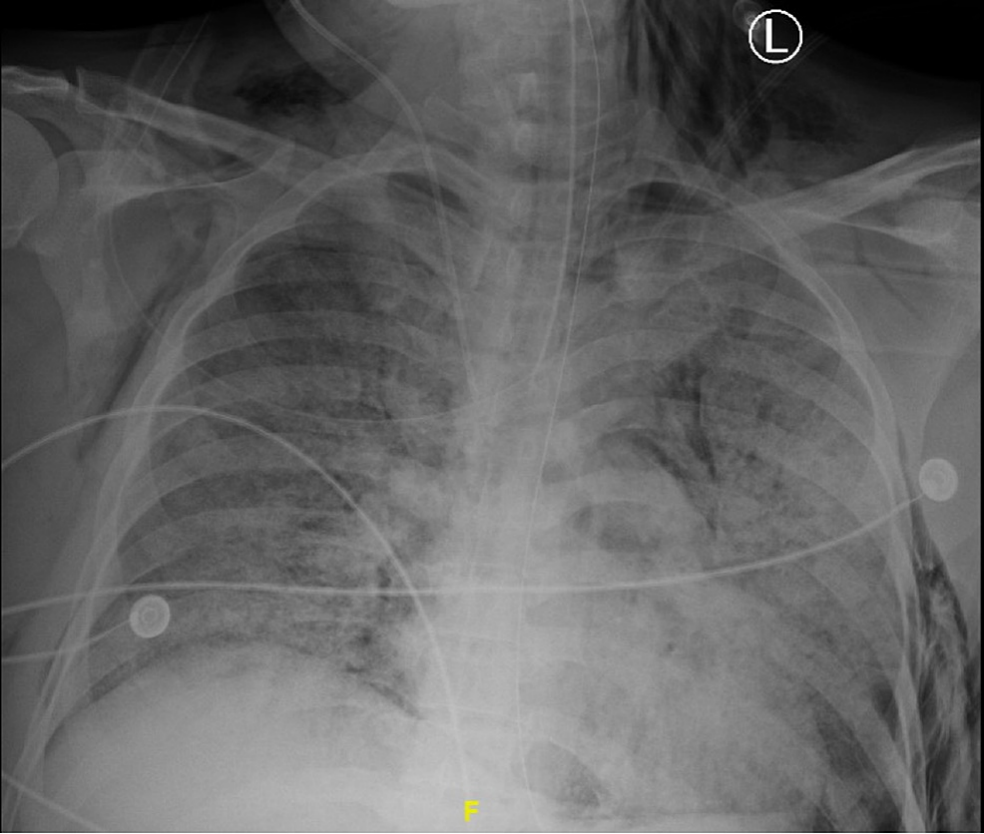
Chest radiograph showing right-sided subcutaneous emphysema and right-sided apical pneumothorax
